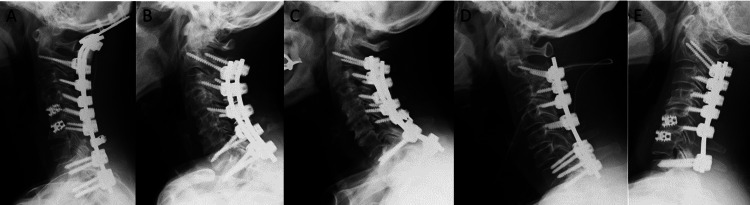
Postoperative neutral lateral cervical radiographs A: Case 1; B: Case 2; C: Case 3; D: Case 4; E: Case 5. Posterior fixation from the occiput or C2 to Th1/Th2 was performed. Cervical kyphosis was corrected in all cases. The numerical identifiers (e.g., Case 1, Case 2, Case 3, etc.) are arbitrary and were created solely for the purpose of referencing specific cases within this article. These identifiers do not correspond to any patient-identifying information.
